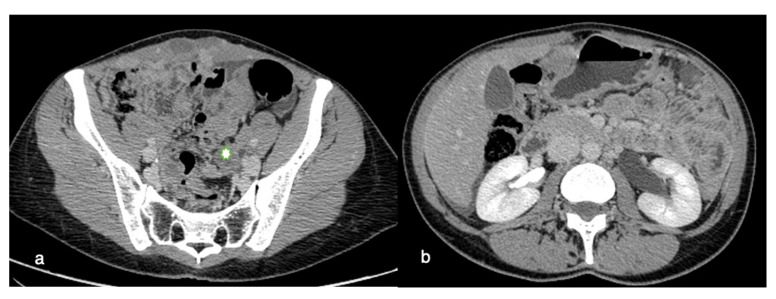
Retroperitoneal involvement. Axial contrast-enhanced CT images show involvement of the left pelvic ureter (a) encased by tumoral tissue (asterisk) associated with left hydronephrosis (b).
